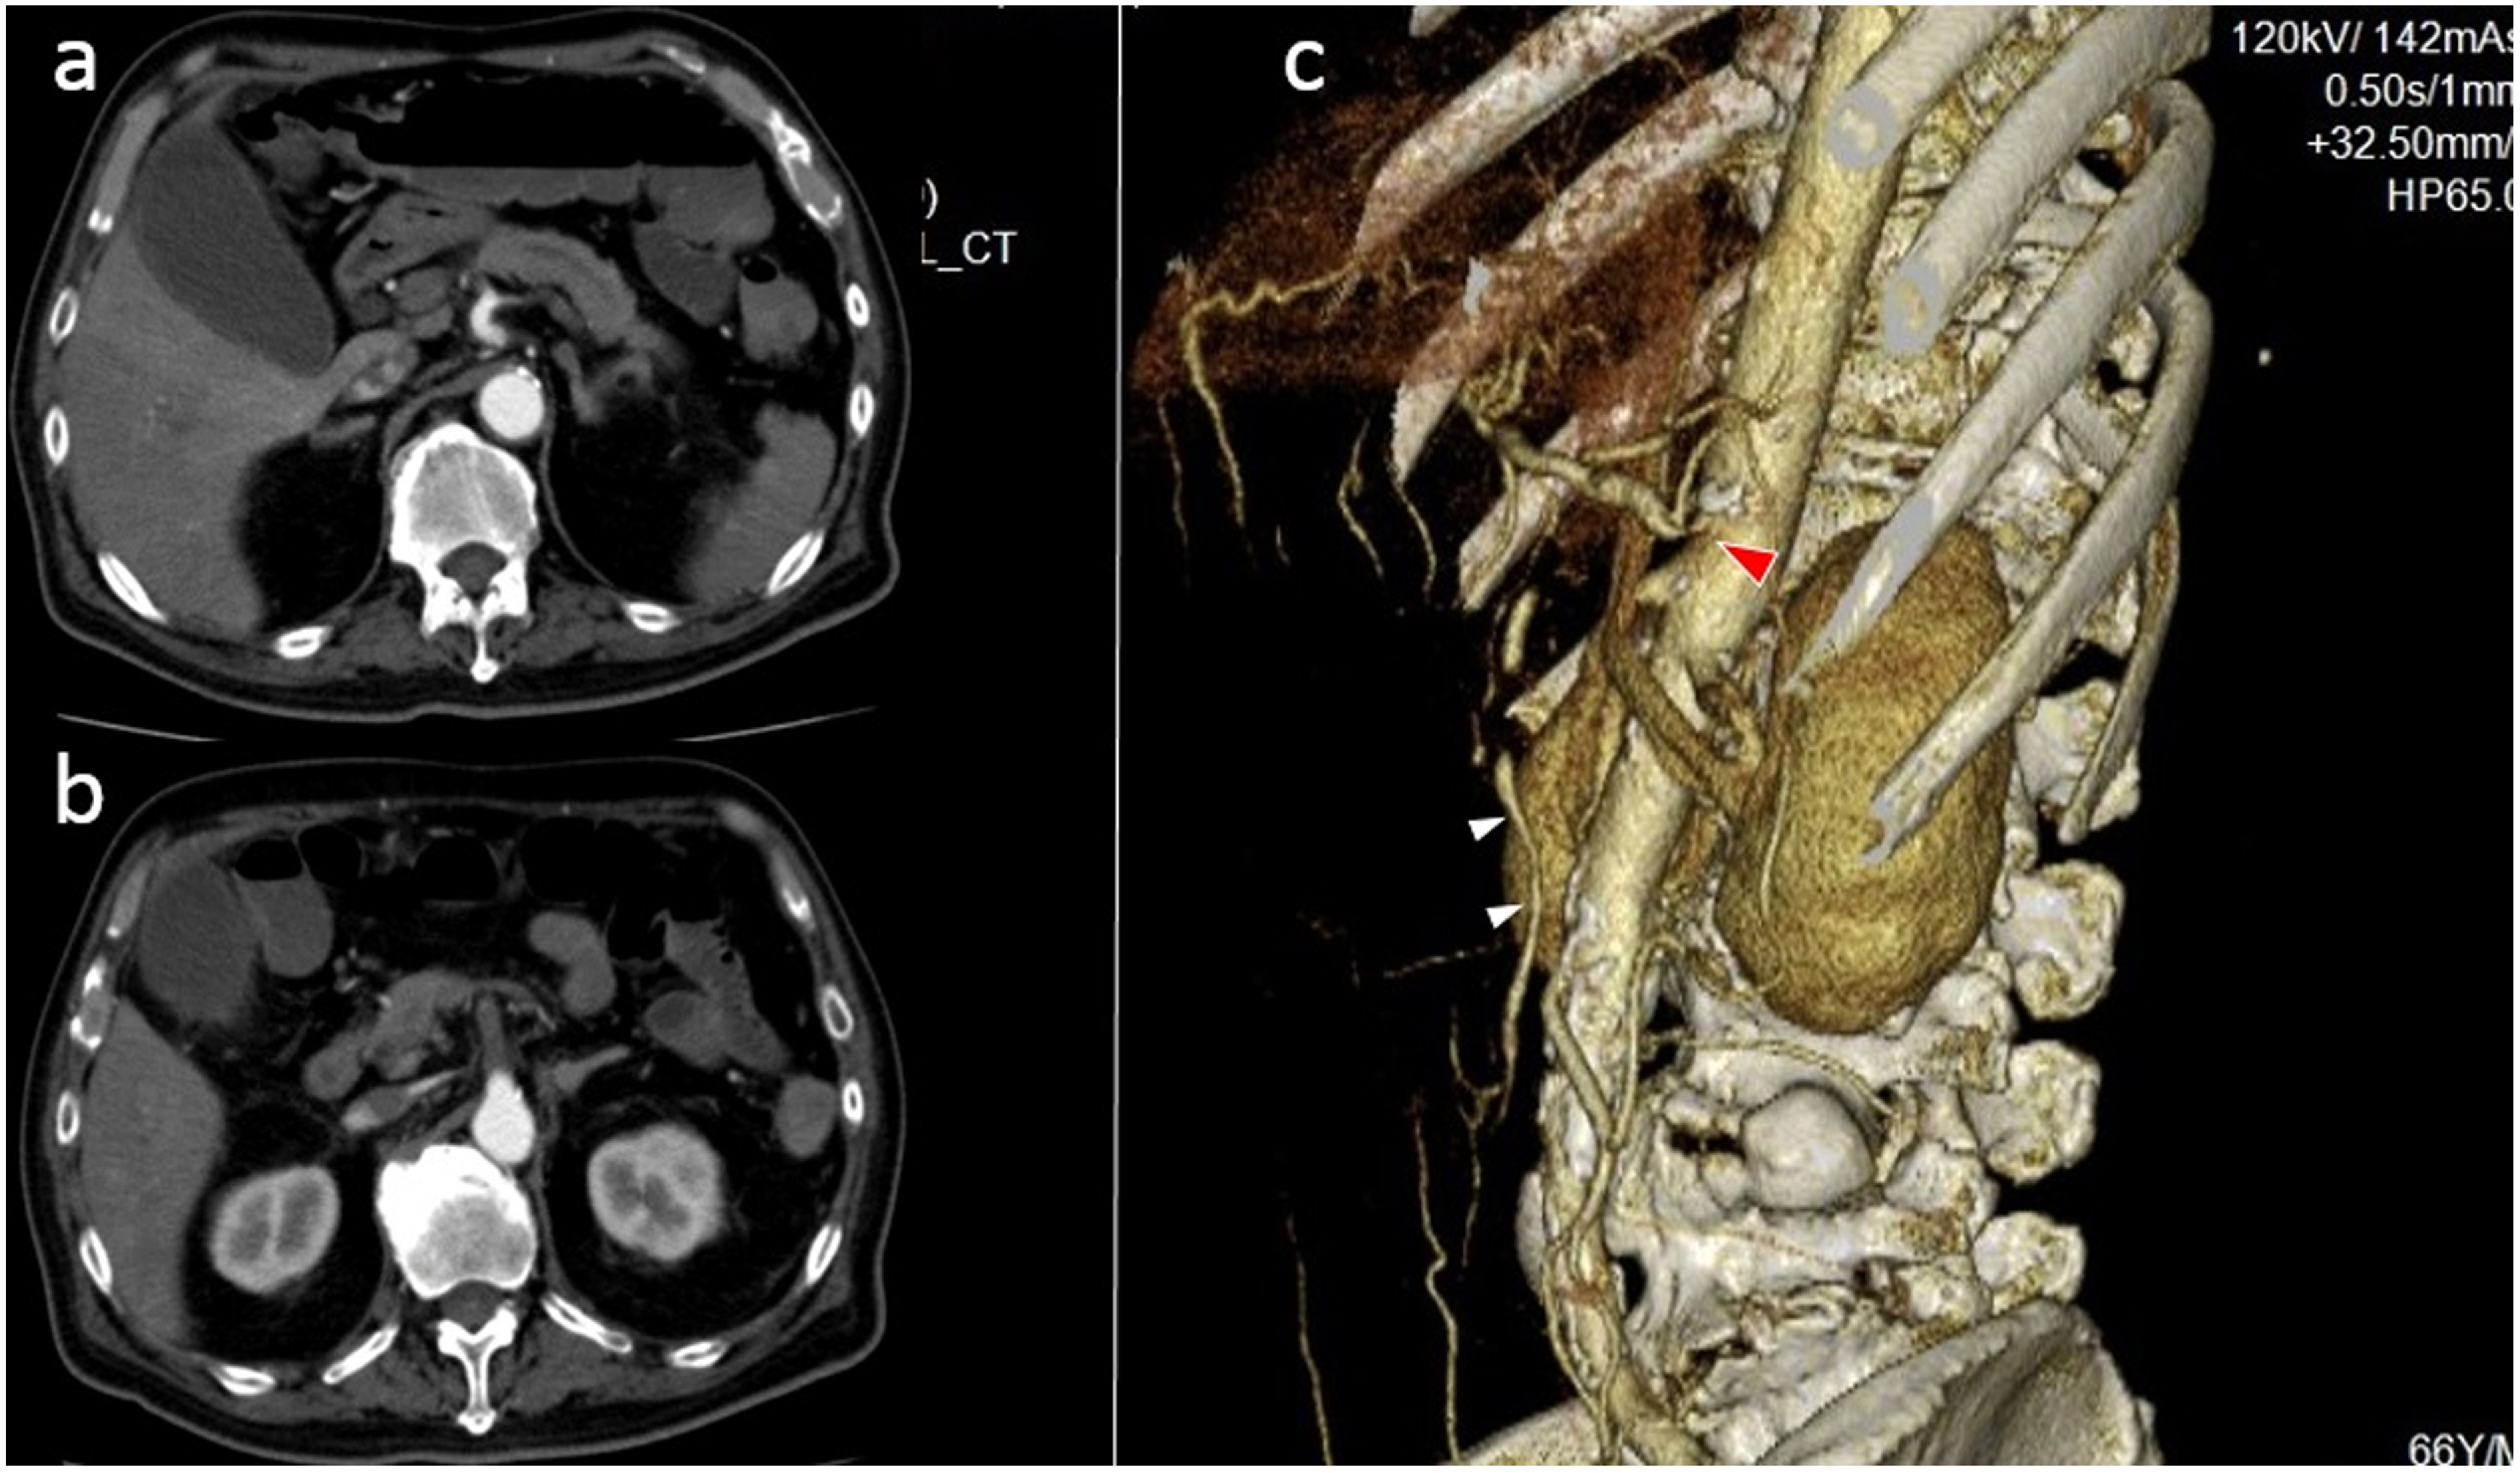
Fig. 1 Preoperative contrast-enhanced computed tomography (CT) images. (a) Proximal part of the celiac artery (CA) shows a significant stenosis due to the median arcuate ligament. (b) Superior mesenteric artery is occluded in the proximal segment. (c) Three-dimensional reconstruction of the CT angiography images represents acute extrinsic stenosis of the CA caused by medial arcuate ligament compression (red arrowhead).
